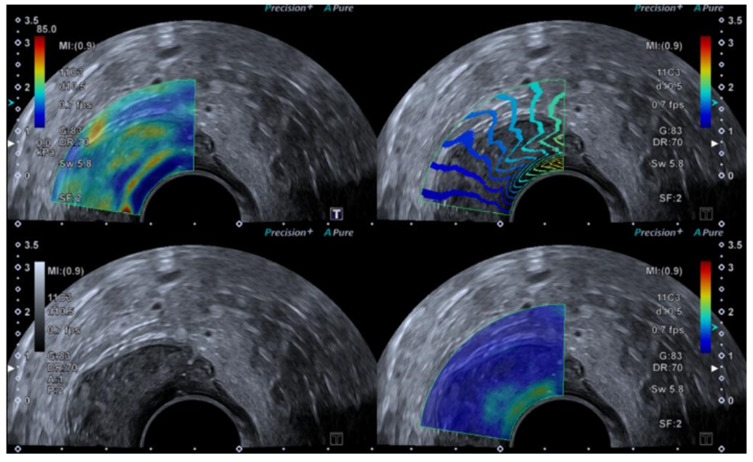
SWE ultrasound showing a polyp in an 85-year-old female.
